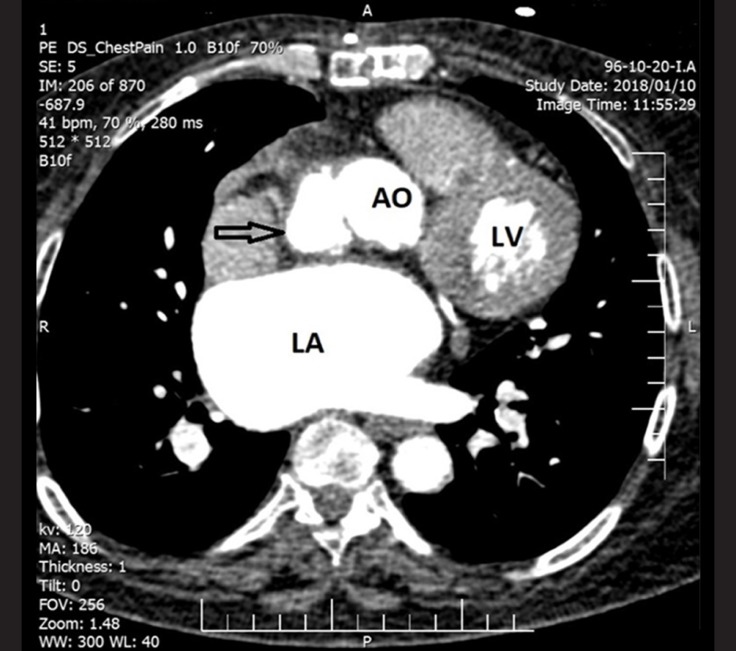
Computed tomography angiography of the aorta in a cross-sectional slice, showing the pseudoaneurysm (arrow) in the posterolateral wall of the ascending aorta
